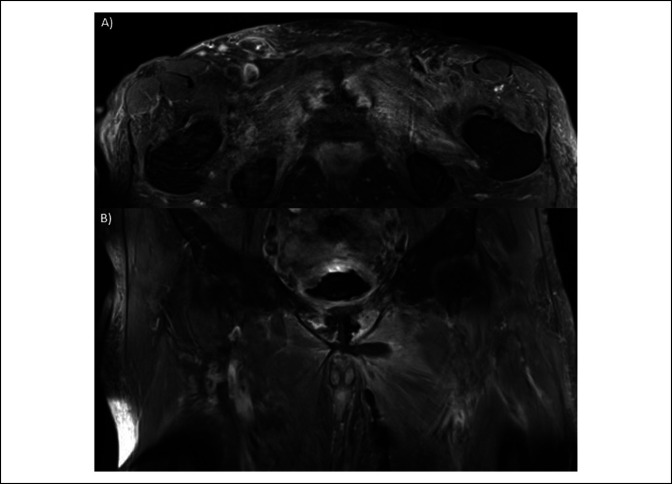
Magnetic resonance imaging of the pelvis showing hyperintense, rim-enhancing lesions along with hypointense pockets of gas on T2 postcontrast imaging. These areas of gas correspond to the areas seen on pelvic CT and are indicative of pubic bone emphysematous osteomyelitis.
